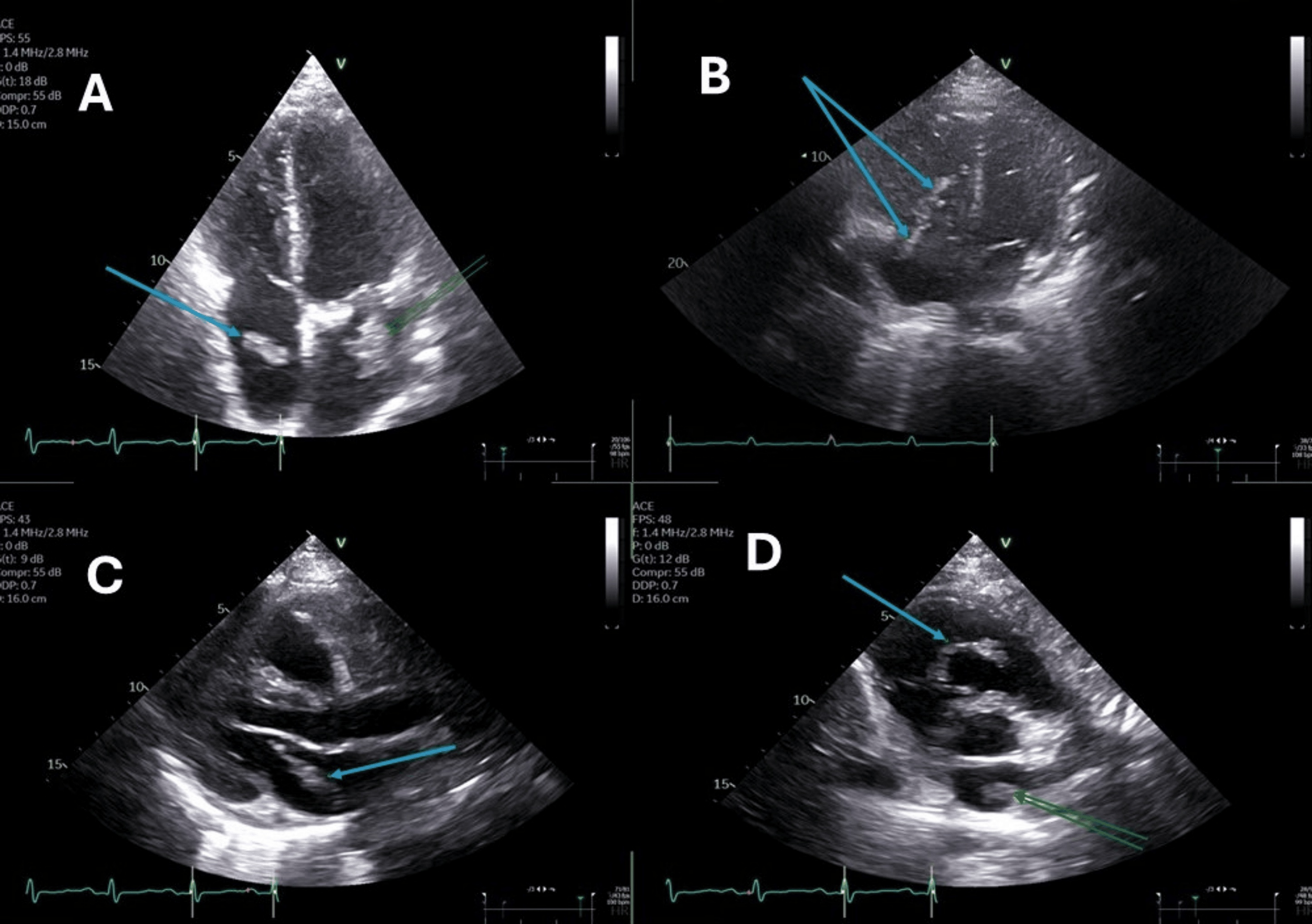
Transthoracic echocardiography (TTE) of bilateral cardiac masses A: Apical four-chamber view (end-systole). Single blue arrow indicates the right atrial mass; double green arrows indicate the left atrial mass. B: Apical four-chamber view (diastole). Double blue arrows highlight the right atrial mass prolapsing from the right atrium into the right ventricle across the tricuspid valve. C: Parasternal long-axis view (diastole). Single blue arrow identifies the left atrial mass. D: Parasternal short-axis view (end-diastole). Single blue arrow shows the right atrial mass in the right ventricular outflow tract; double green arrows indicate the left atrial mass.
